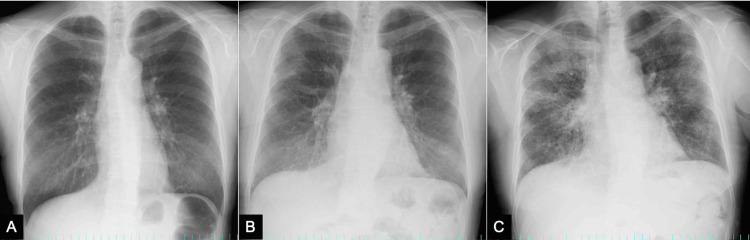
A series of chest X-rays (A) Chest X-ray one year before admission, (B) Chest X-ray on admission showing bilateral peripheral ground-glass opacities, (C) Chest X-ray on day nine showing the deterioration of lung opacities
